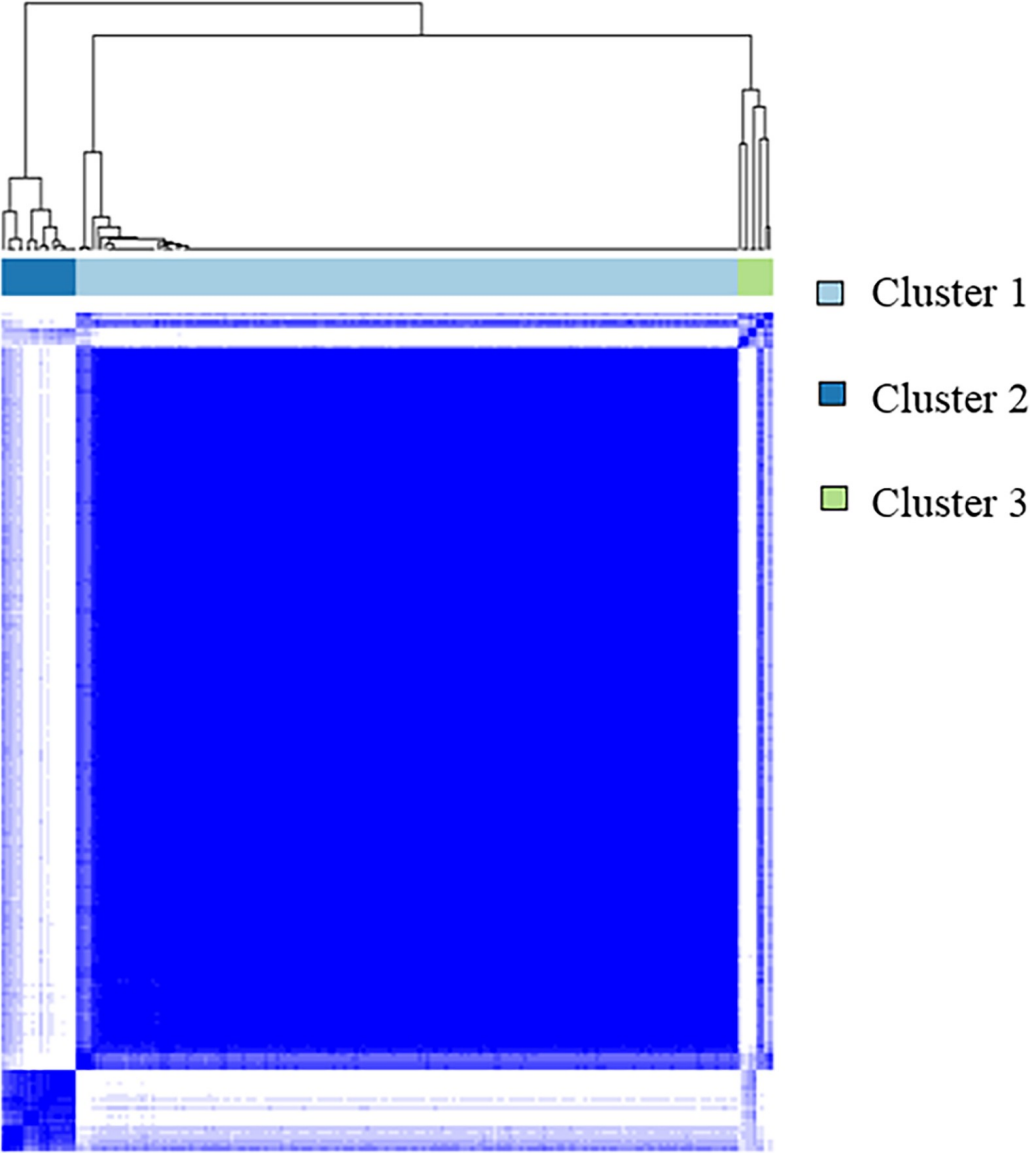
Consensus Cumulative Distribution Function (CDF) Pot using quantitative CT and clinical values.
